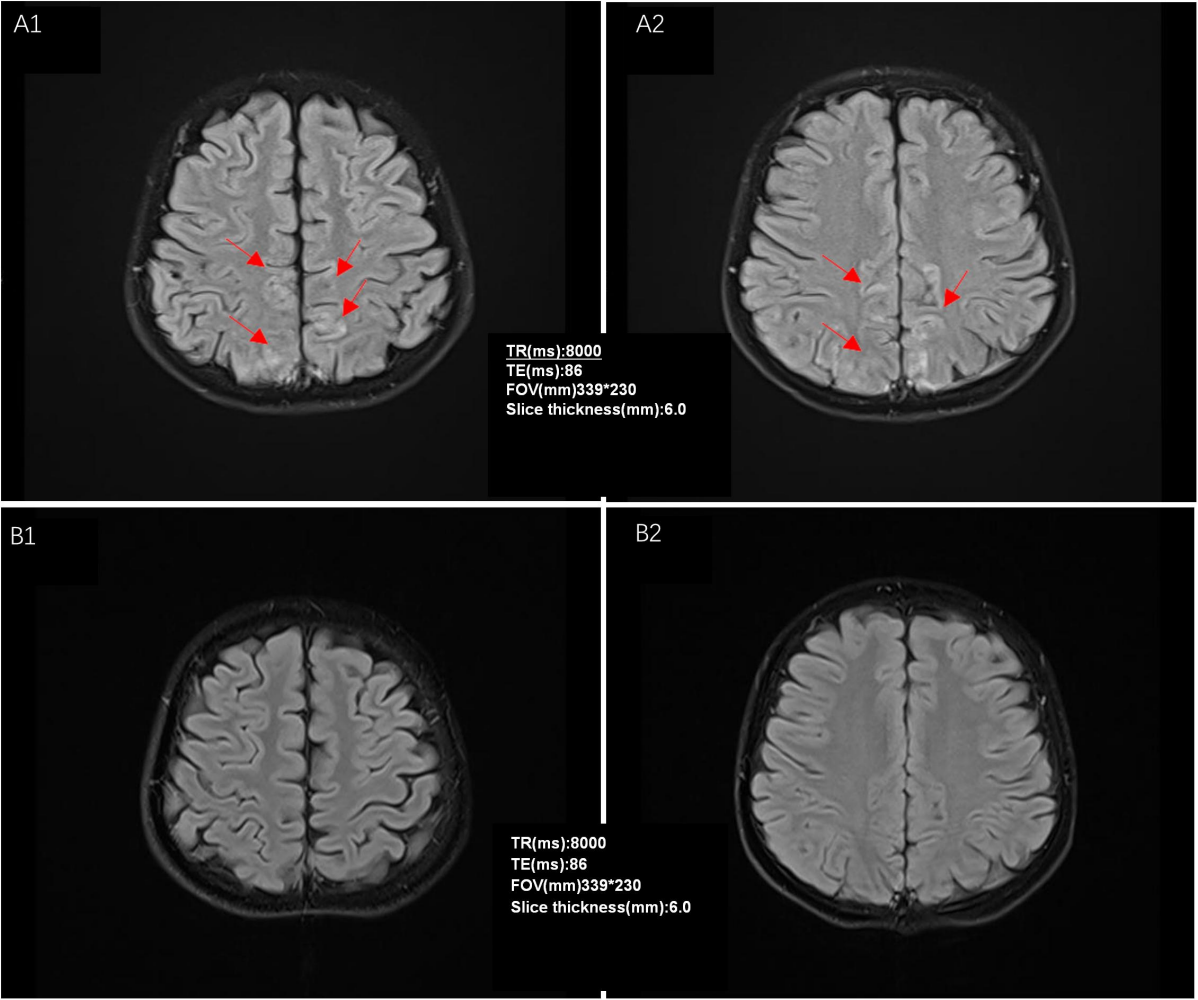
Patient's head imaging assessment. A1 and A2 are bilateral parietal lobe cortical and subcortical patchy slightly high signal shadows on T2-FLAIR images at the onset of the disease. B1 and B2 in the figure show that the abnormal signal shadows in the bilateral parietal lobe cortex and subcortex on T2-FLAIR images have completely disappeared when reexamined 3 months after the onset of the disease.
